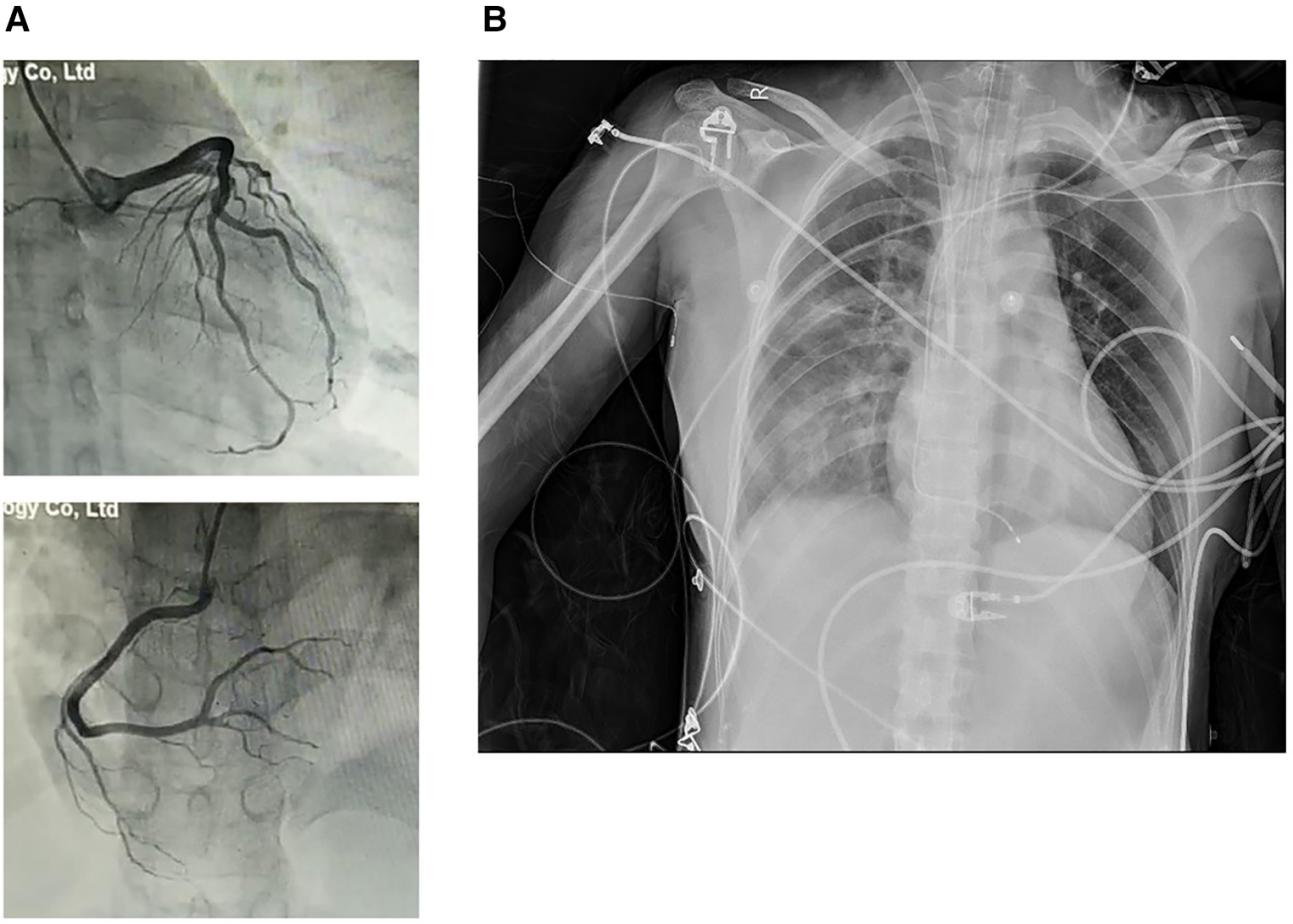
(A) CAG excluded coronary artery stenosis. (B) Chest radiography revealed enlarged cardiac size and a lung infection.
